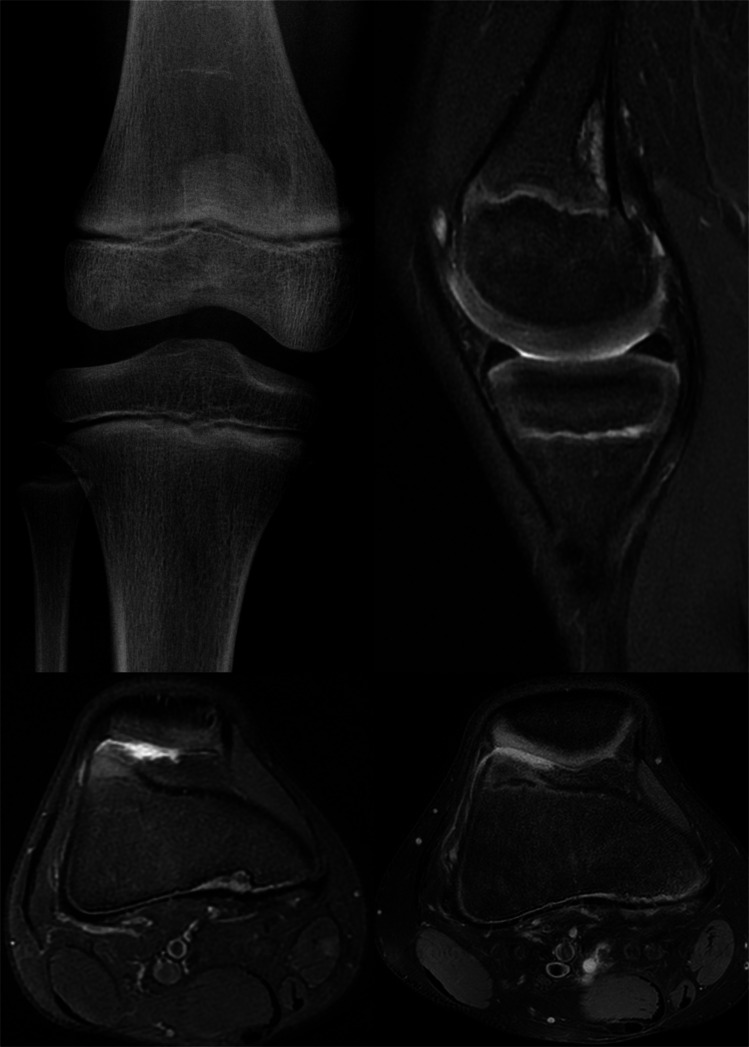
Eight-year-old male with distal femoral cortical irregularity (DCFI). AP knee radiograph (top left) demonstrates a well-defined eccentrically located lucency within the medial distal femoral metaphysis with a peripheral sclerotic margin. Sagittal and axial PDFS (top right and bottom left) images reveal a corresponding area of signal change with a peripheral low signal margin, underlying the medial head of gastrocnemius enthesis. Interval axial PDFS image 4 years later (bottom right) demonstrate resolution of the DFCI which is now difficult to demonstrate from the normal distal femoral metaphyseal stripe of vascular subperiosteal fibrous tissue
